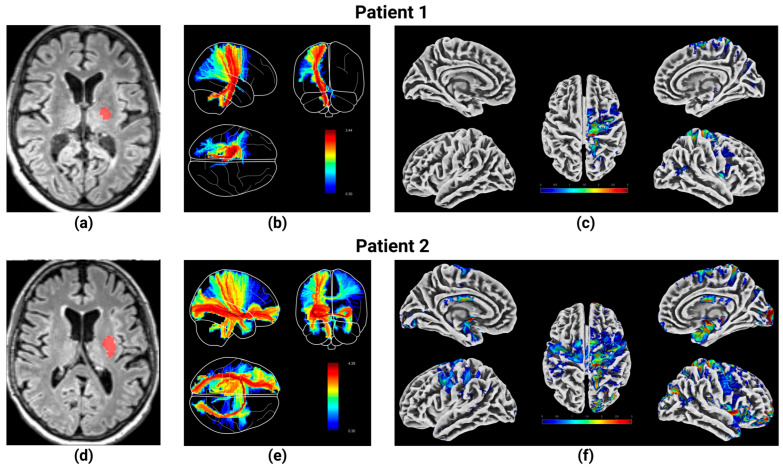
Representative MRI example from two patients with left-hemispheric subcortical ischemic stroke. Top row: unilaterally affected patient 1; bottom row: bilaterally affected patient 2. (a,d) Original FLAIR image with the outlined lesion in red. (b,e) Functional connectivity network originating from the lesion. (c,f) Cortical regions affected by the lesion and its connectivity.
